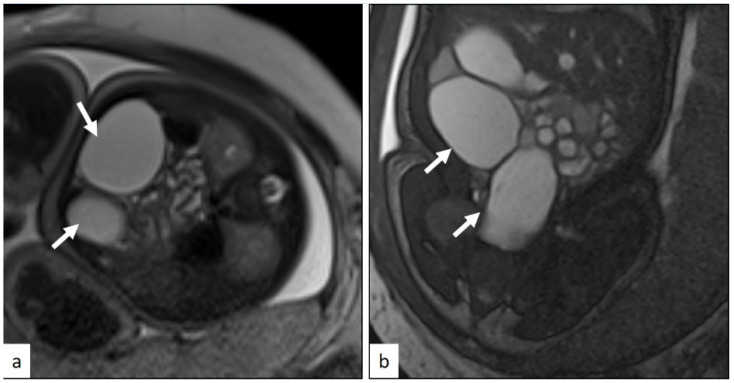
TruFISP axial (a) and coronal (b) planes MRI images demonstrate thin-walled cystic lesions in the midline and left side (arrows). There was no bowel obstruction. Kidneys were normal. The diagnosis was thought to be ovarian cysts. Postnatal diagnosis confirmed the ovarian cysts.
